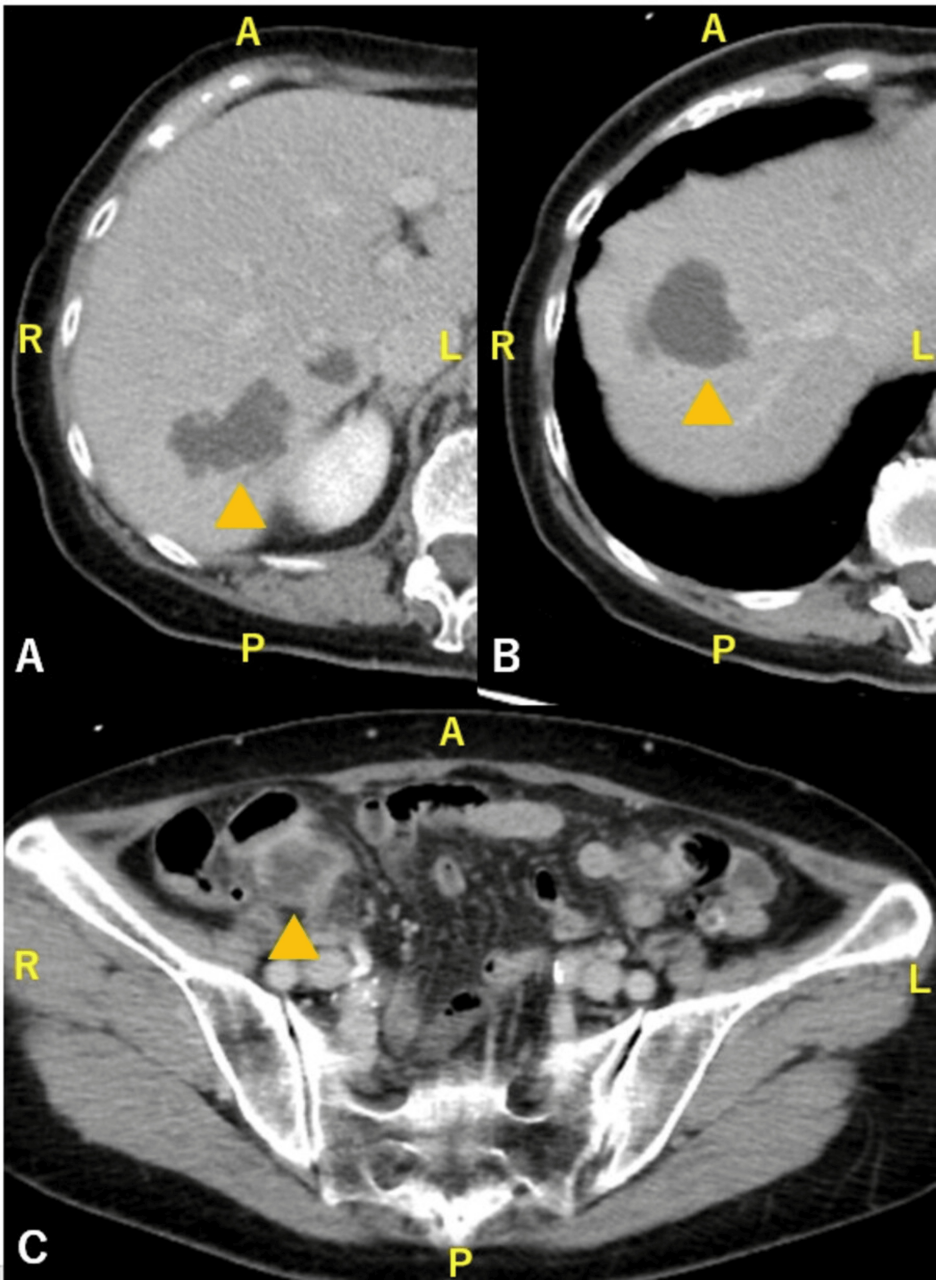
Contrast-enhanced CT at admission (A, B) Multiple abscesses are seen in the right hepatic lobe (arrowheads). (C) An additional abscess is noted dorsal to the terminal ileum (arrowhead) CT: computed tomography
